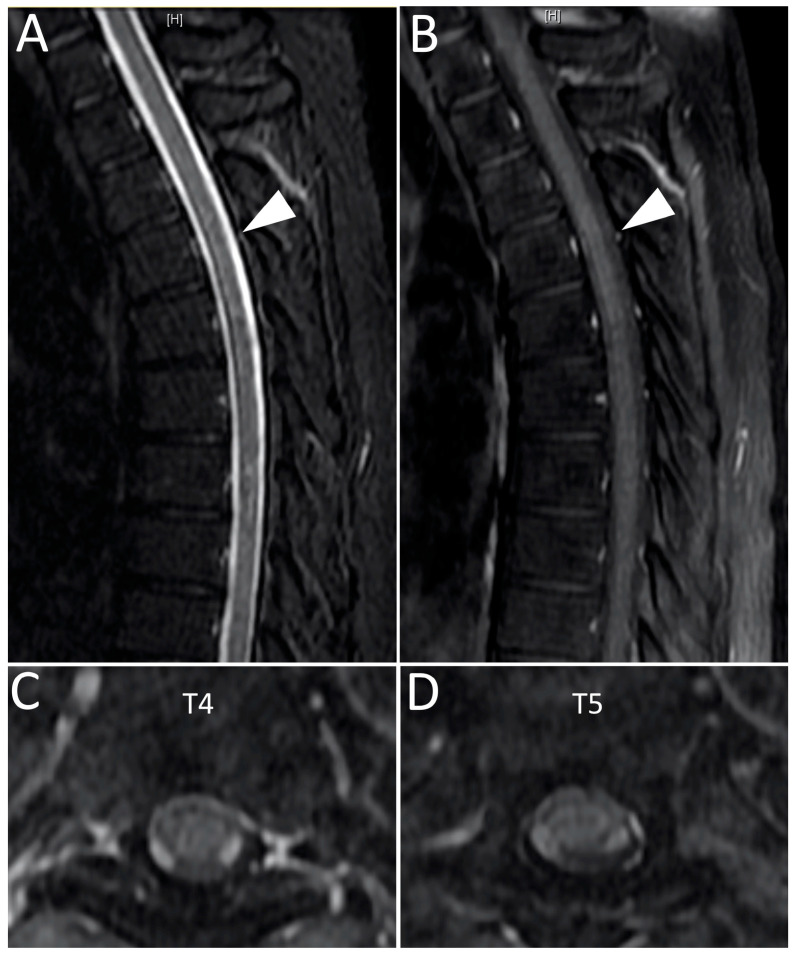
The follow-up MRI 16 weeks after vaccination of the thoracic spine on the T2-weighted short tau inversion recovery scan (A) and post-contrast T1-weighter fast spin echo scan (B) revealed the recovery of the previous lesion over T1-T6 (indicated by arrowheads). The axial view of the post-contrast T1-weighter fast spin echo scan over T4 (C) and T5 (D) also had similar findings.
